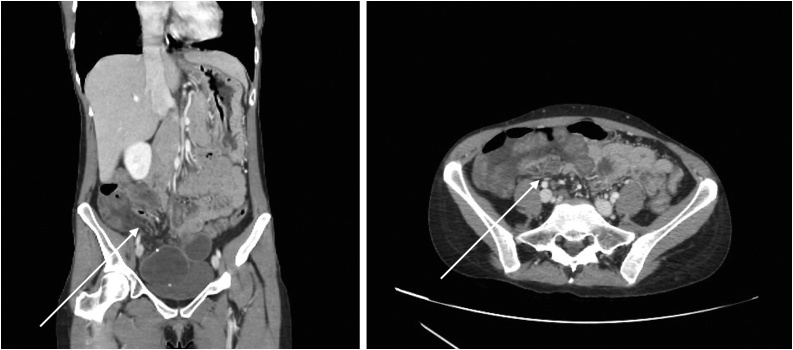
CT image reveals a closed dialed loop localized to the right of the uterus (arrow).
